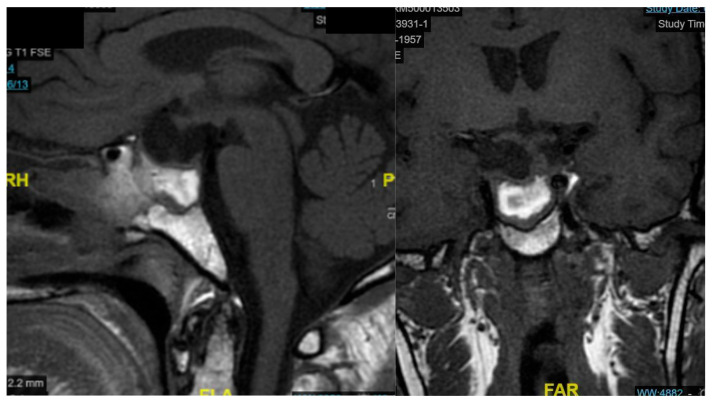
Sagittal and coronal MRI one month after surgery.
